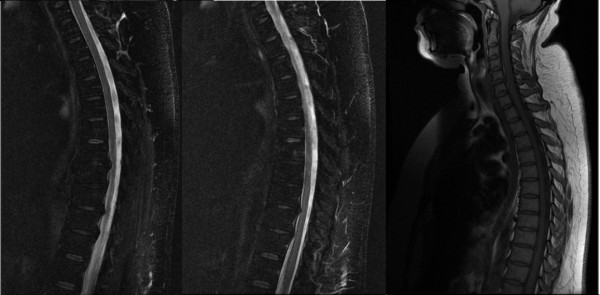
Magnetic resonance imaging scans of the spinal cord acquired the day after readmission. Fast short tau inversion recovery (T2-weighted and T1-weighted, (A) and (B), respectively) and T1-weighted fast spin echo (C) sagittal images of the spinal cord are shown: minor degenerative disease can be observed but their is no cord compression from disc or abscess and no obvious intrinsic signal change.
